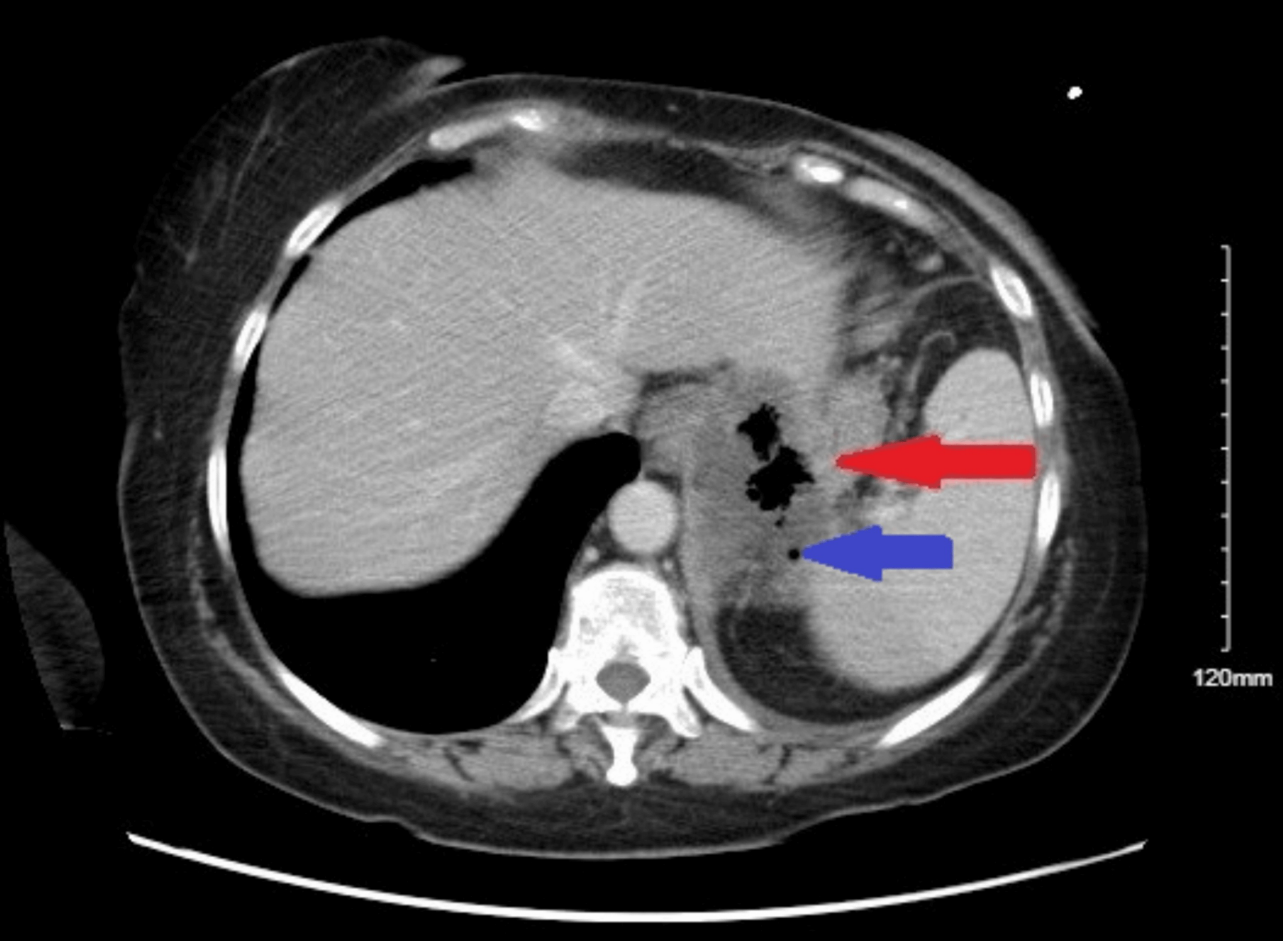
CT abdomen/pelvis with contrast showing a GEJ mass. GEJ mass measuring up to 6.7 cm (red arrow). Distal gastric fundus ulceration is adjacent to the GEJ, with concern for perforation (blue arrow) CT: computed tomography; GEJ: gastroesophageal junction
